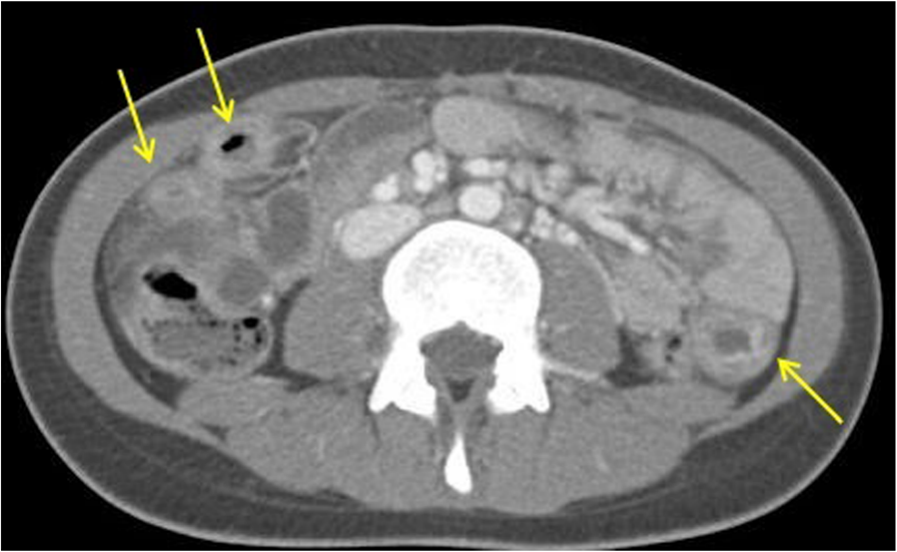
Computed tomography of the abdomen showing an edematous bowel. This image shows multifocal areas of bowel wall thickening, mesenteric edema, and vascular engorgement in jejuno-ileal loops (yellow arrows).
